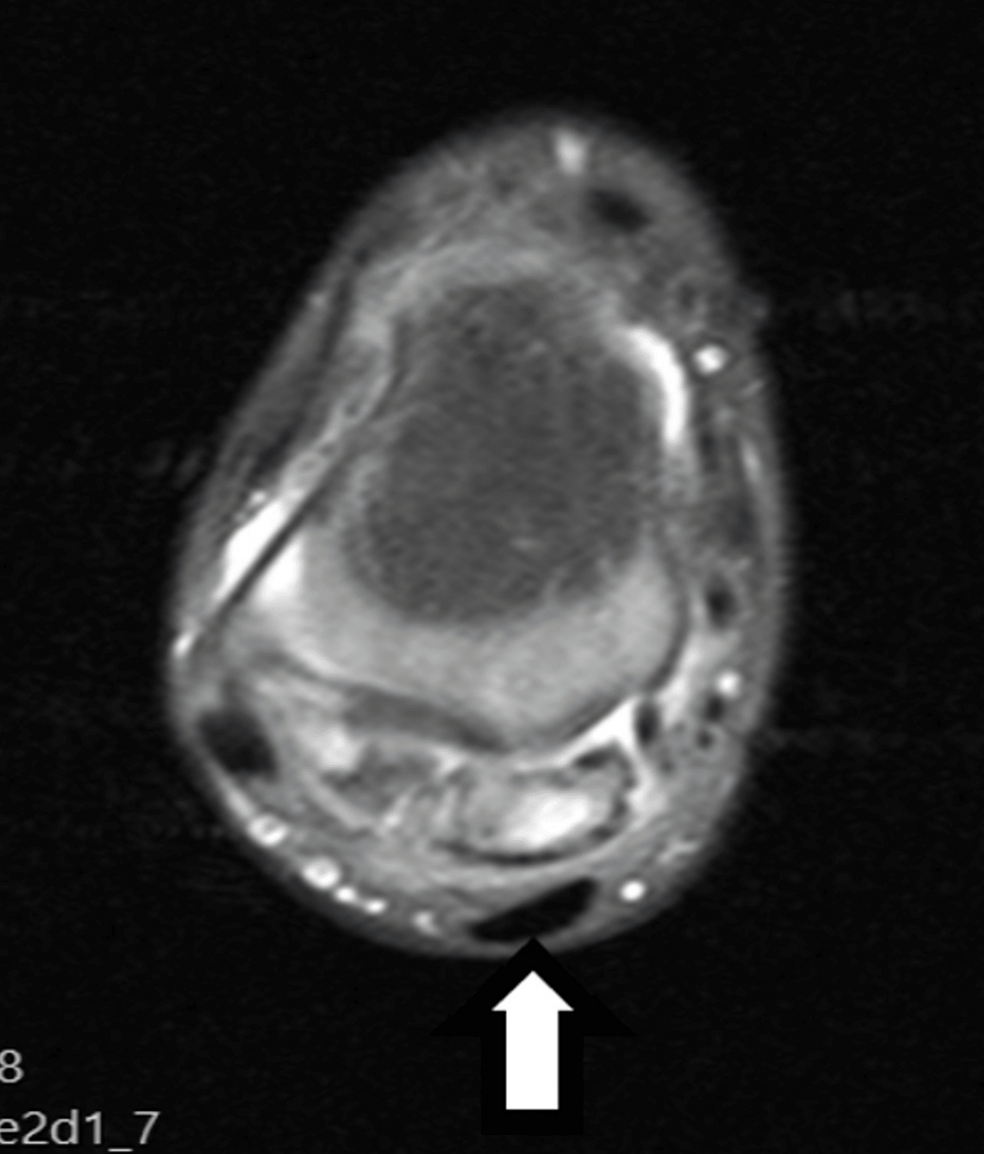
MRI scan of the right ankle, axial proton density (PD) fat saturated. Proton density (PD) sequence with fat suppression in the axial plane also demonstrates the relationship of the mass with the adjacent anatomic structures.
